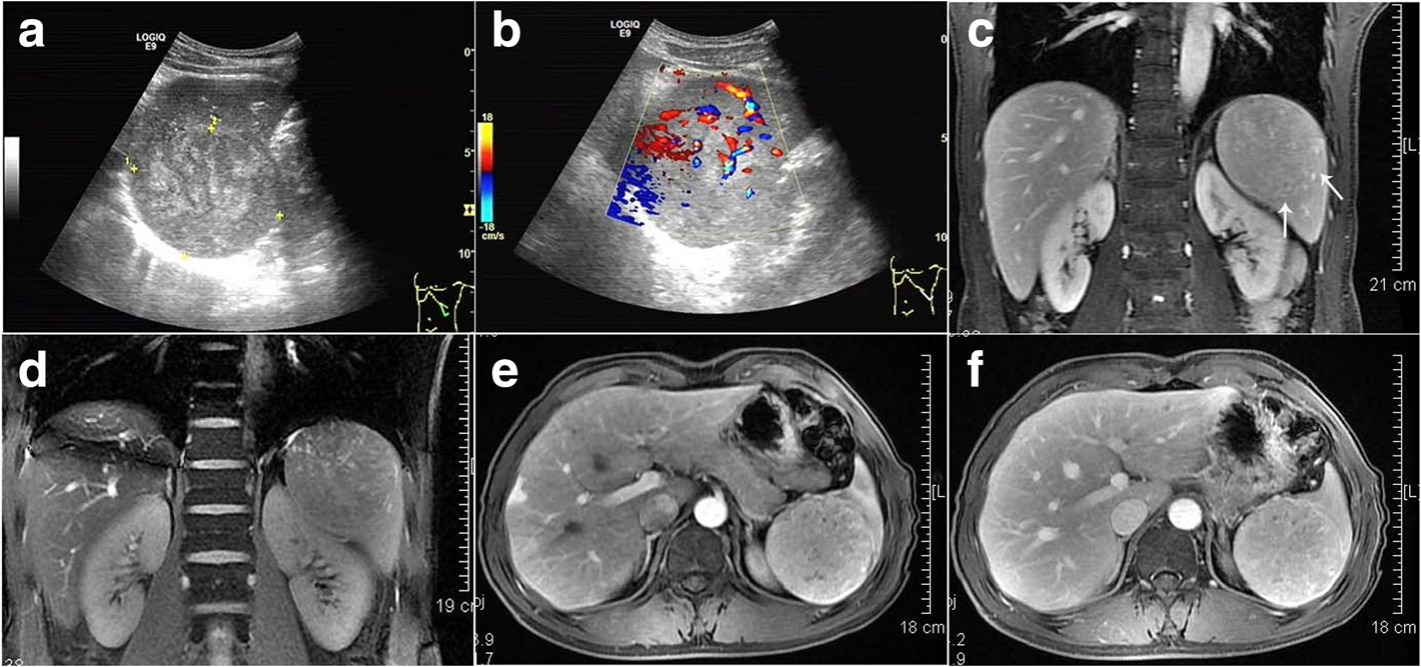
A splenic mass was found incidentally in a 40-year-old man. The abdominal ultrasound image (a) showed a round mixed echogenicity mass in the spleen, in which inhomogeneous echogenicity with patchy hyperechoic and iso-echoic foci were noted. Internal color Doppler flow was also observed (b). Magnetic resonance imaging (MRI) displayed a nearly iso-signal lesion (arrow) compared to the splenic parenchyma both on T1-weighted (c) and T2-weighted (d) images. The mass demonstrated relatively homogenous enhancement to the same degree as that of the splenic parenchyma on arterial phase (e) and delayed phase (f)
